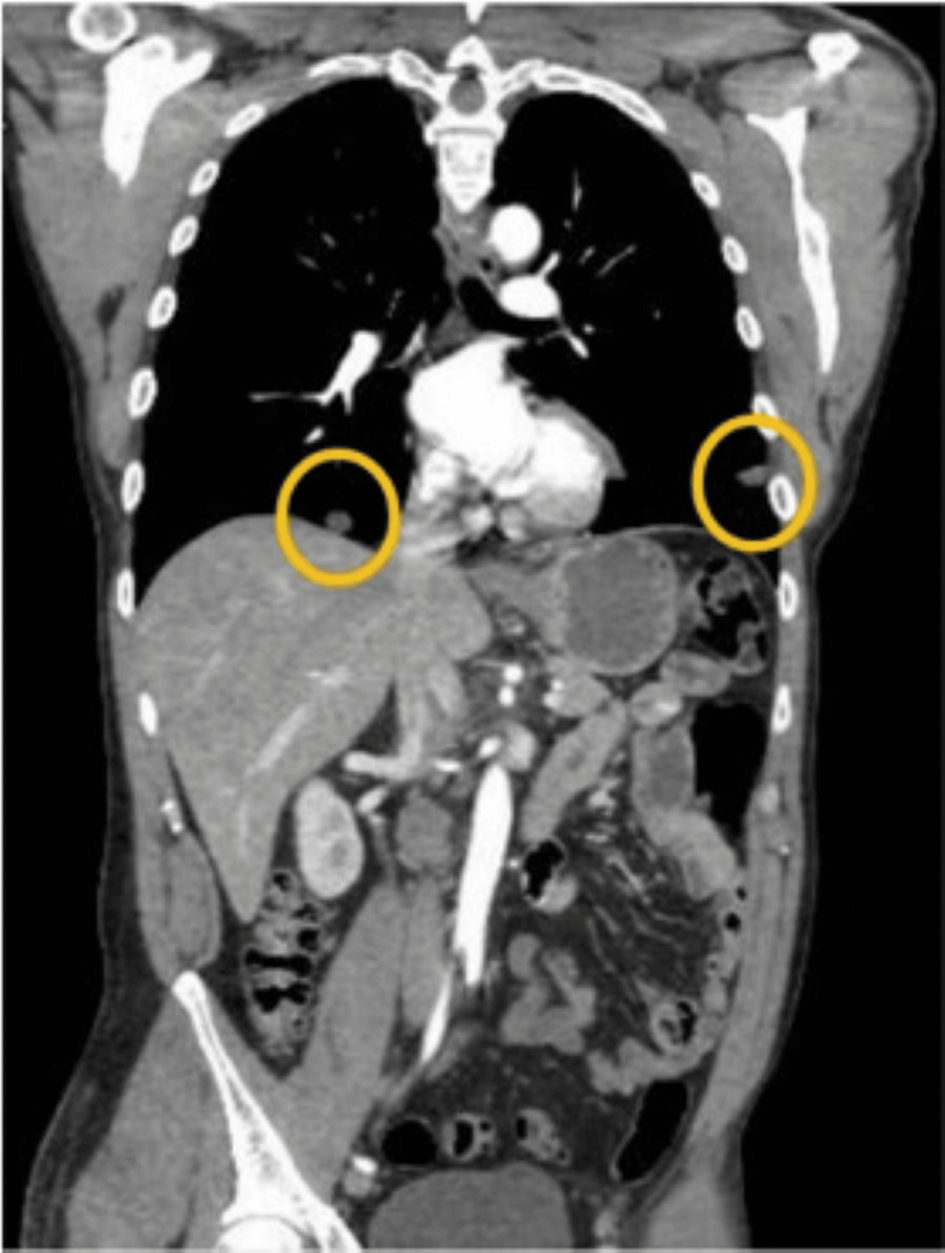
CT chest CT angiography of the chest demonstrating bilateral pulmonary metastases
